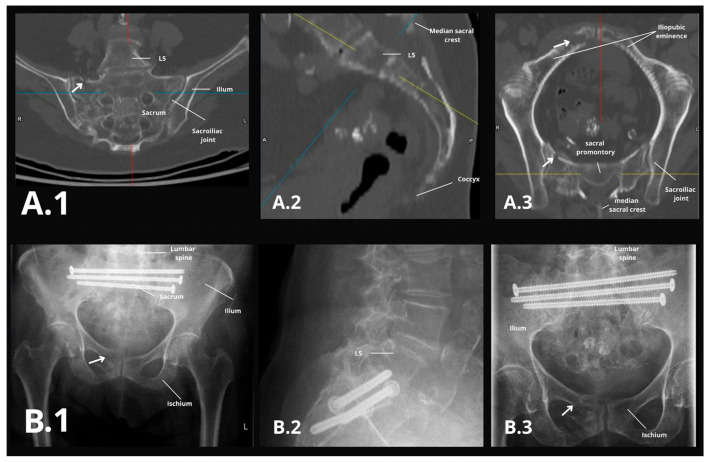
Preoperative and postoperative imaging. (A) The firs row shows preoperative axial pelvic CT scan demonstrating a type IIIc sacral insufficiency fracture (Fragility Fractures of the Pelvis [FFP] classification). (A.1) Coronal CT image showing a sacral fracture. The white arrow indicates the fracture line through the sacrum. (A.2) Sagittal CT reconstruction and (A.3) axial CT image of the pelvis showing the extent of the fracture. (B) The second row shows postoperative radiographies showing transsacral screw fixation with two screws at S1 and one screw at S2 levels. (B.1) Anteroposterior (AP) pelvic X-ray, (B.2) lateral lumbar spine X-ray, and (B.3) AP pelvic X-ray showing fixation of the sacrum after 1 month of follow-up. Blue, red and yellow lines have no semantic significance.
